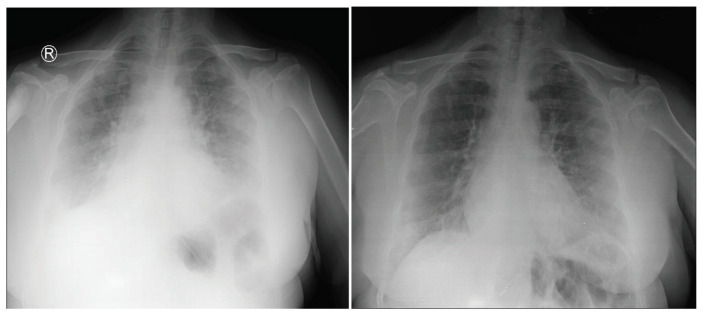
The chest radiographs of a patient who used aluminum salts, taken at 2 days interval.
